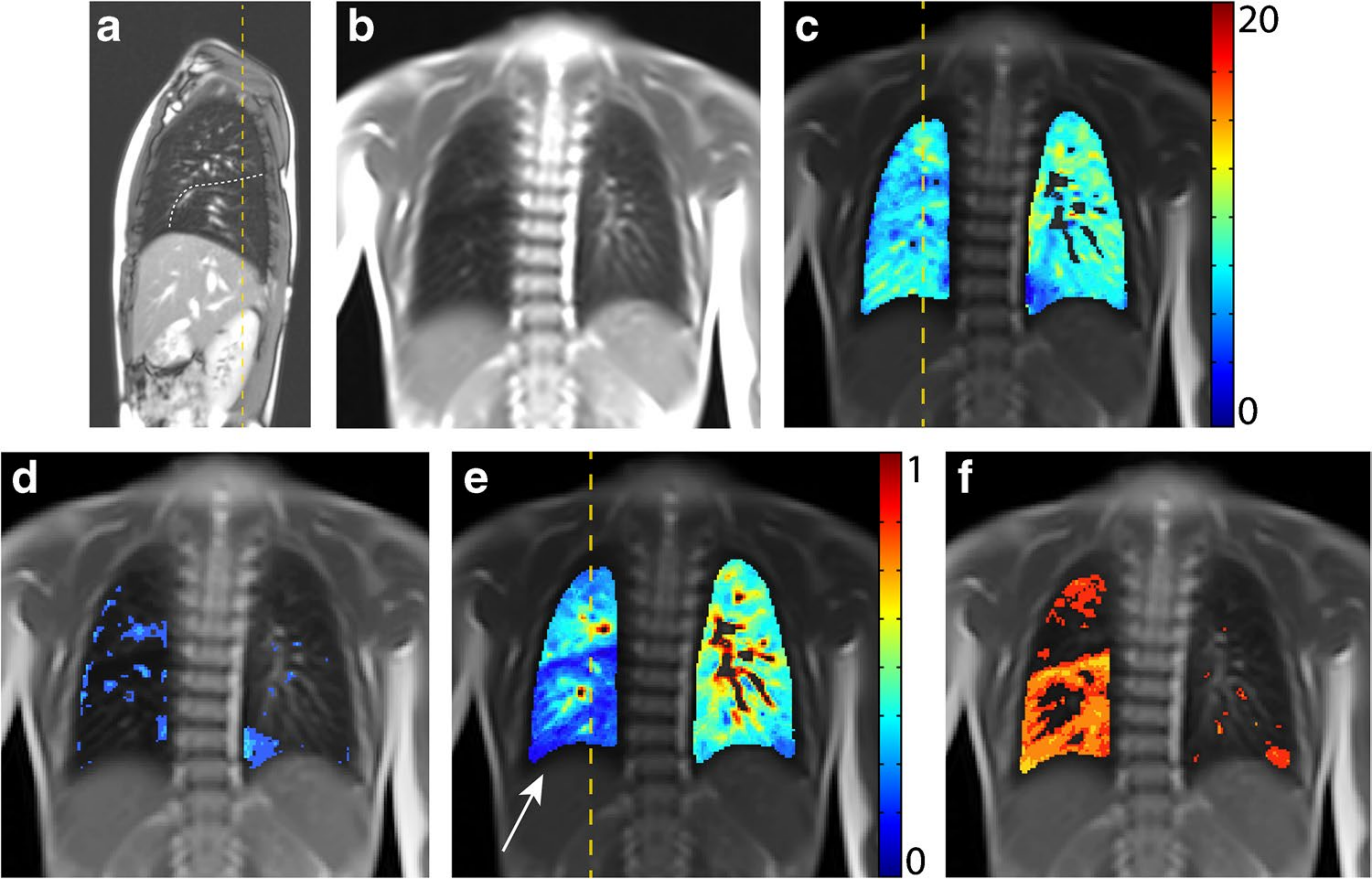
A 7-year-old girl following lobectomy of the right lower lobe for hybrid lesion (intralobar bronchopulmonary sequestration, CPAM type 1) and an increased lung clearance index of 8.37 turnovers. a A sagittal T2-weighted image (repetition time [TR]/echo time [TE] 3.3/1.3 ms) and (b) a coronal T1/2-weighted image (TR/TE 1.5/07 ms) demonstrate expansion of the middle lobe into the cavity of the resected right lower lobe. c-f Coronal functional magnetic resonance imaging maps: (c) coronal fractional ventilation, (d) fractional ventilation defect percentage (brighter blue areas represent reduced function), (e) relative perfusion and (f) relative perfusion defect maps (brighter orange areas represent reduced function) show a fractional ventilation defect percentage (13.1%) within the norm and an increased perfusion defect percentage (26.1%) in the area of the former right lower lobe, to which region the middle lobe has expanded (e) (*white arrow*). *Yellow dashed lines* indicate plane levels. *White dashed line* indicates lung fissure for better visibility. *CPAM* congenital pulmonary airway malformation
